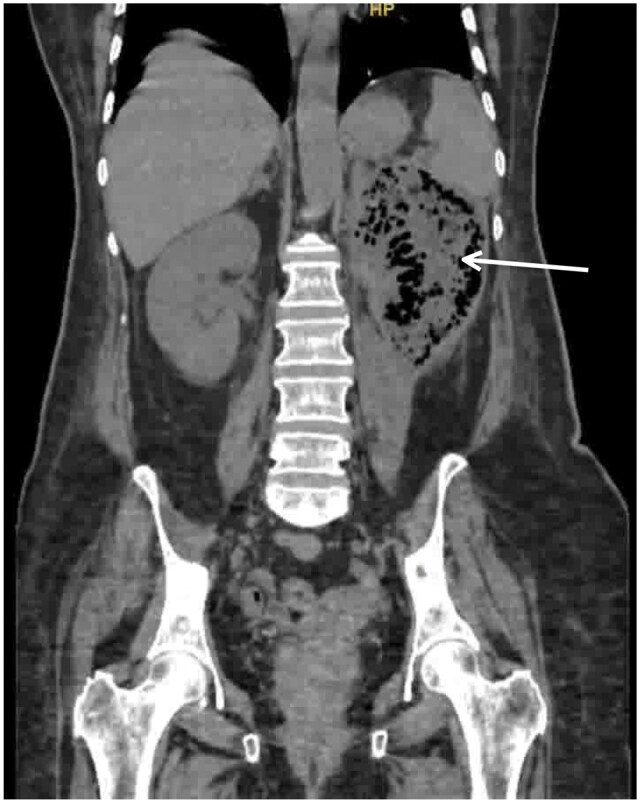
Coronal non-contrast CT image showing air-containing collection involving the left kidney with extension into the perinephric space (arrow).
